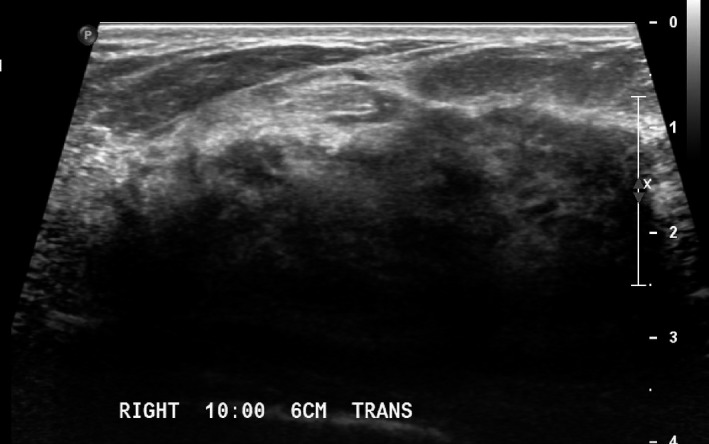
Second‐look right breast ultrasound was performed after MBI. At 10:00, 6 cm from the nipple, there is a vague ill‐defined hypoechoic area with shadowing, corresponding with the area of MBI uptake and palpable concern
